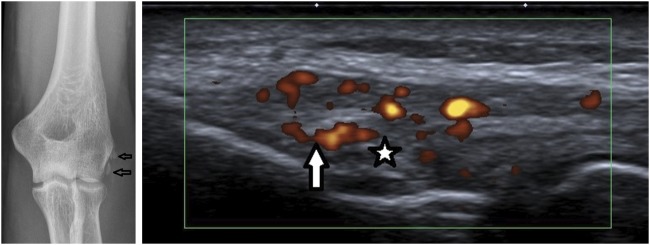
Left elbow X-ray and US: calcification and hyperaemia at the common extensor tendon Anteroposterior X-ray of the left elbow shows two amorphous calcifications (arrows) at the lateral epicondyle of the left humerus. US image of the common extensor tendon and the calcification (star) and hyperemia (arrow) on colour Doppler around the calcification
